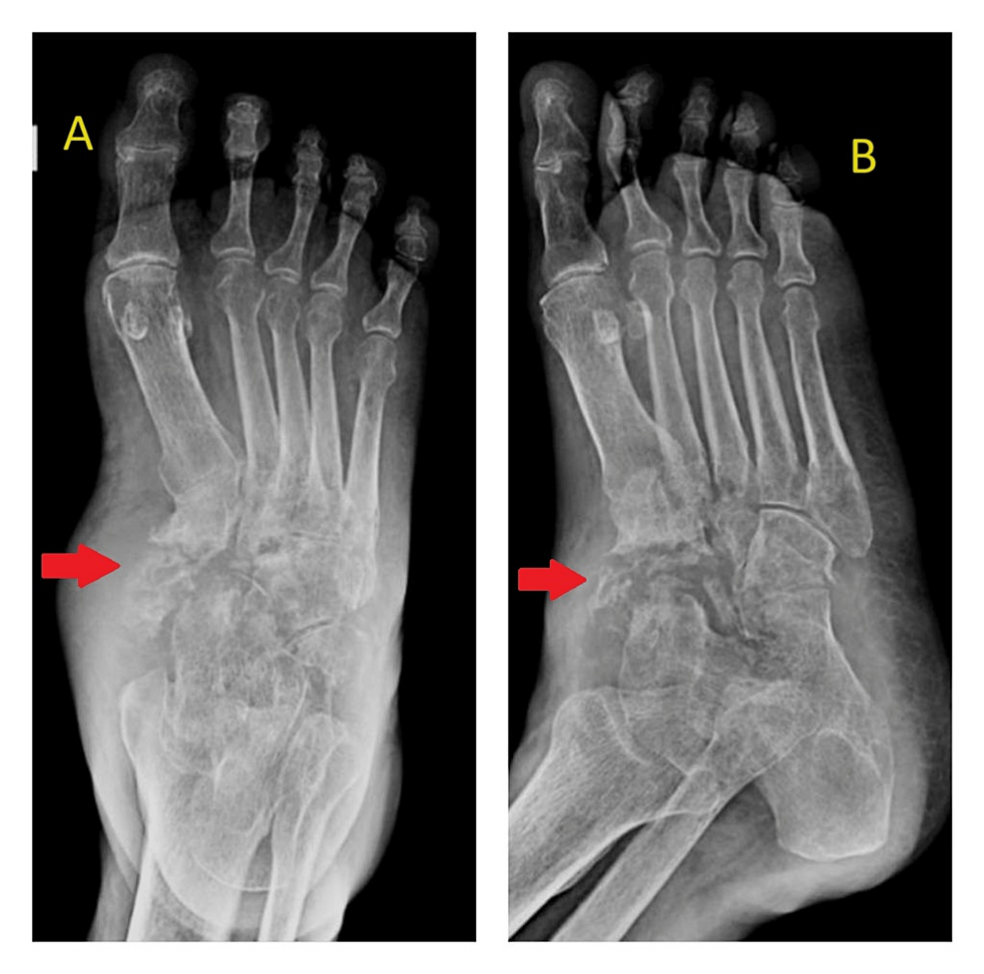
X-ray of right foot anteroposterior (A) and oblique (B) views showing generalized osteoporosis, periarticular soft tissue edema, subchondral erosions, or destructive lesions in the tarsal bones and base of the metatarsals suggestive of osteomyelitis changes; absence fracture seen.
